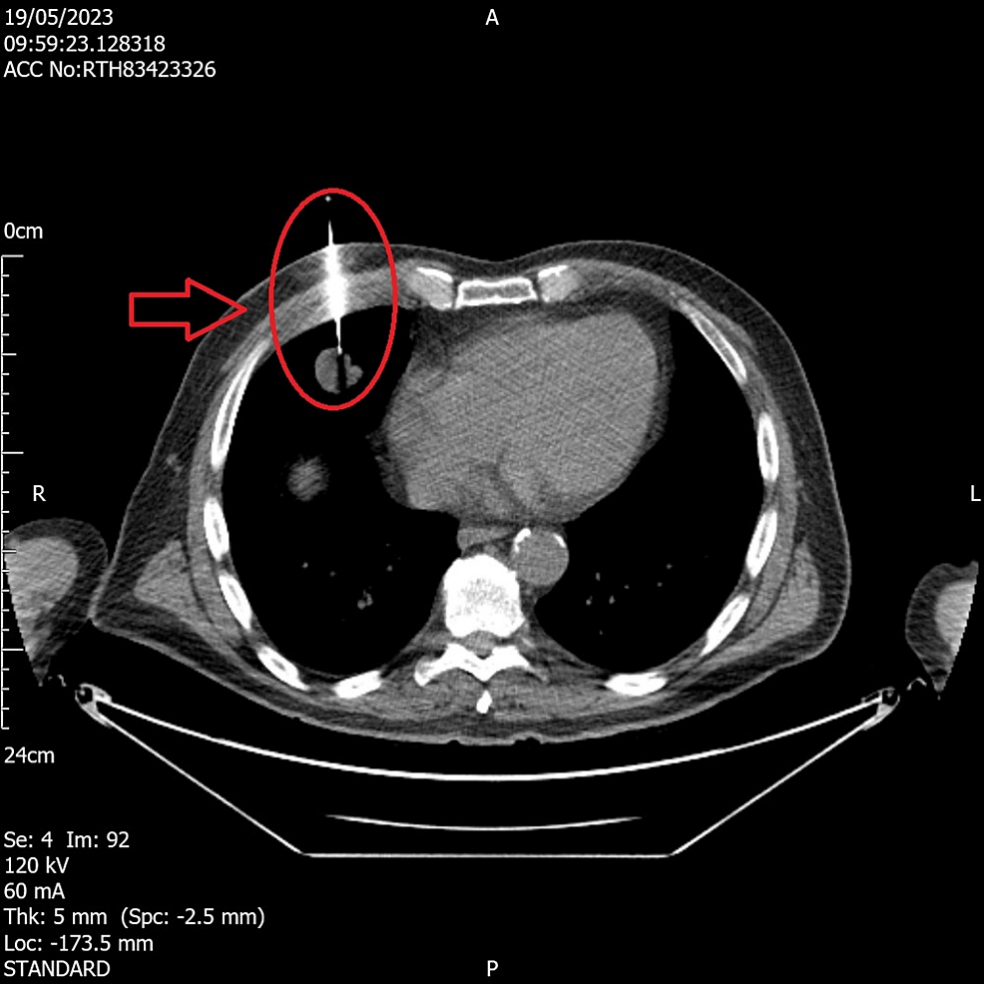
CT-guided biopsy of pulmonary nodule
